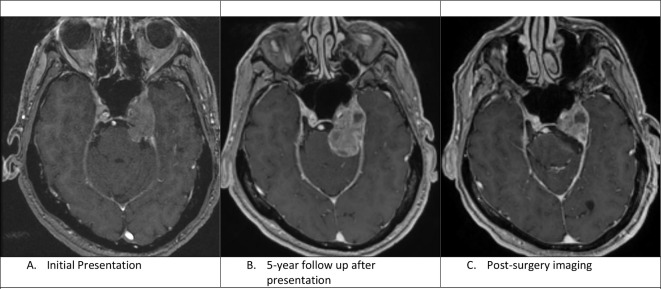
A: Axial C+ T1-weighted image shows an enhancing mass centred in the left cavernous sinus with mild displacement of the medial left temporal lobe. B: Axial C+ T1 weighted image shows interval enlargement of the prepontine component of the mass. There are also some new cystic changes within the mass. C. Axial C+ T1-weighted image shows residual enhancing mass lesion after resection of the cisternal component of the mass.
